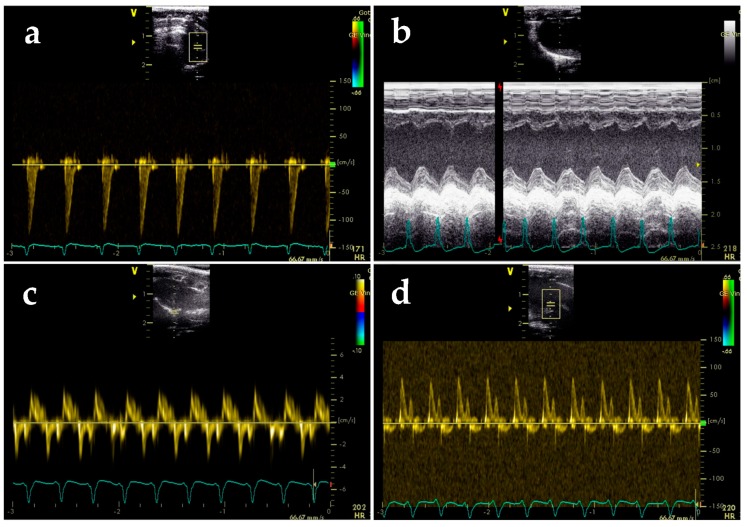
Representative images of echocardiographic experiments. (a) Left-ventricle outflow tract (LVOT), visualized by Doppler echocardiography; (b) parasternal long axis, M-mode image of left ventricle; (c) 3-chamber view, mitral annular velocities, tissue Doppler mode; (d) mitral inflow velocities, visualized by Doppler echocardiography.
